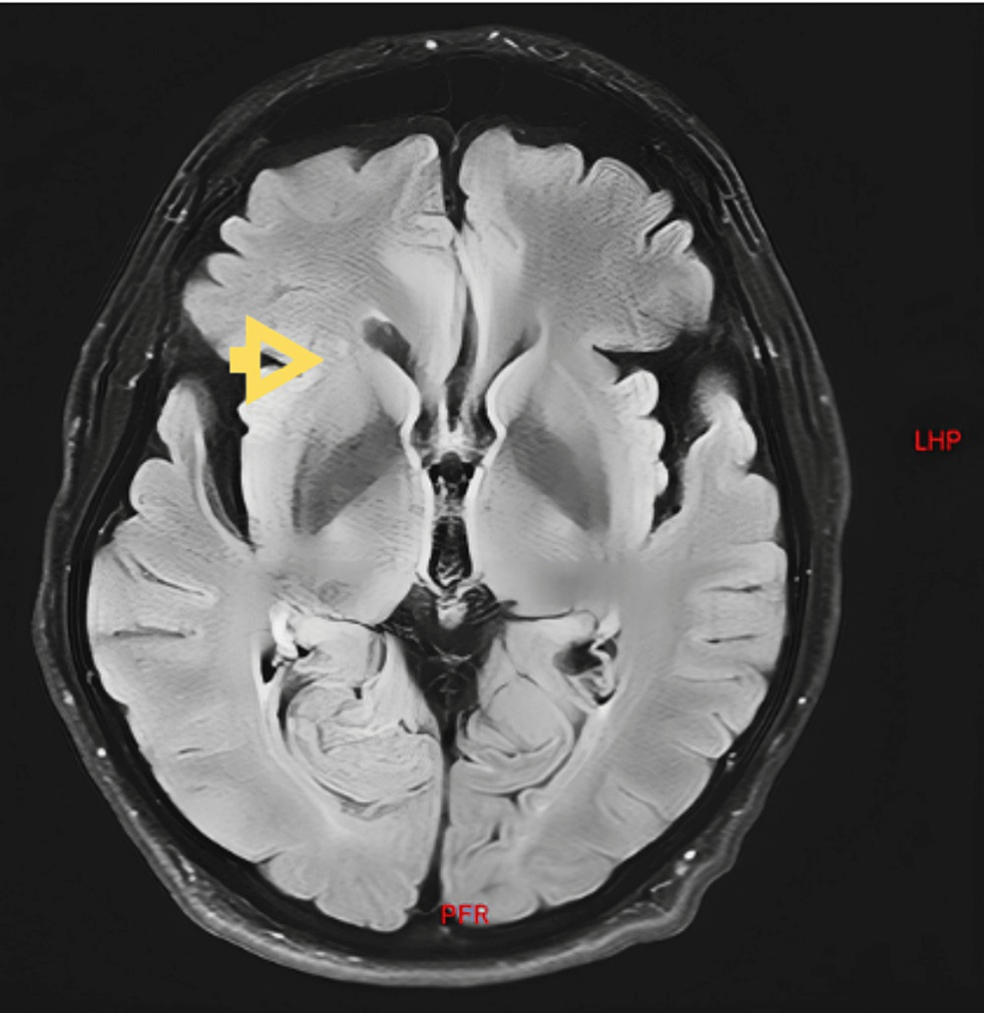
Brain MRI in T1/FLAIR sequence in axial plane In this image, a punctate subcortical right frontal hyperintensity is observed.
